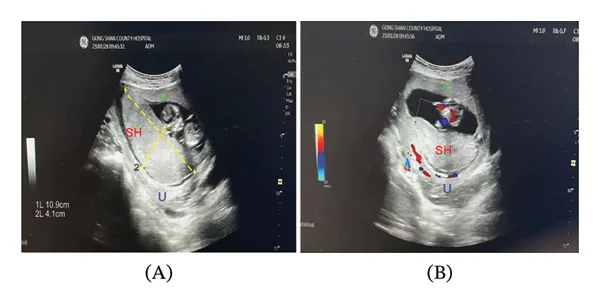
Color Doppler ultrasound images of the fetus and placenta at 12 weeks plus 6 days of gestation. (A) The fetus and placenta were observed by LOGIQ S8 (GE, USA) using a 5.0‐Hz transducer. It was revealed that the placenta was implanted on the anterior uterine wall. A well‐demarcated, hypoechoic zone measuring 109 × 41 mm^2^, representing the largest cross‐sectional area, was identified beneath the gestational sac chorionic plate. Fetal examination is normal. The two yellow dashed lines are the measurement lines for subchorionic hematoma. (B) The blood flow of subchorionic hematoma was also observed with LOGIQ S8. No discernible intraparenchymal or perilesional vascularity was observed within the hematoma region. P represents the placenta; SH represents the subchorionic hematoma; U represents the uterus.
